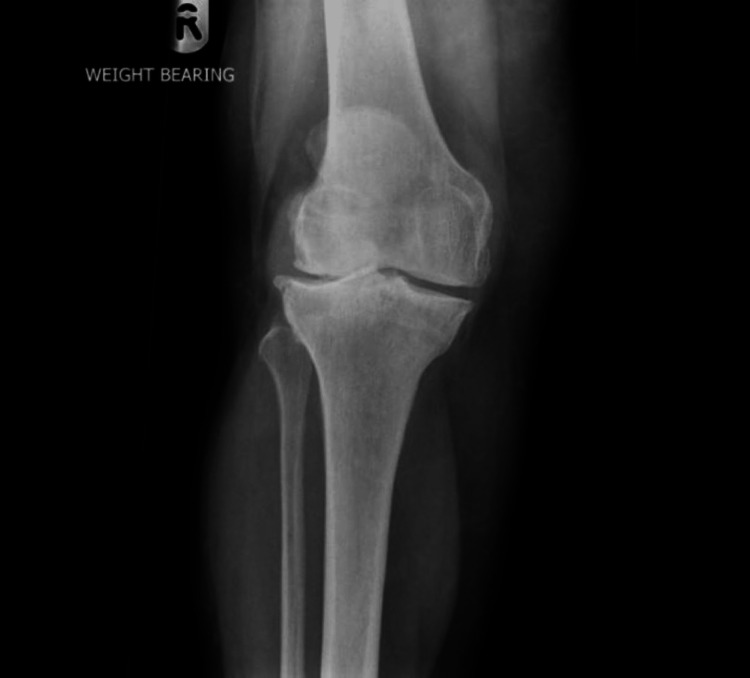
Preoperative X-ray, AP view AP: anteroposterior
